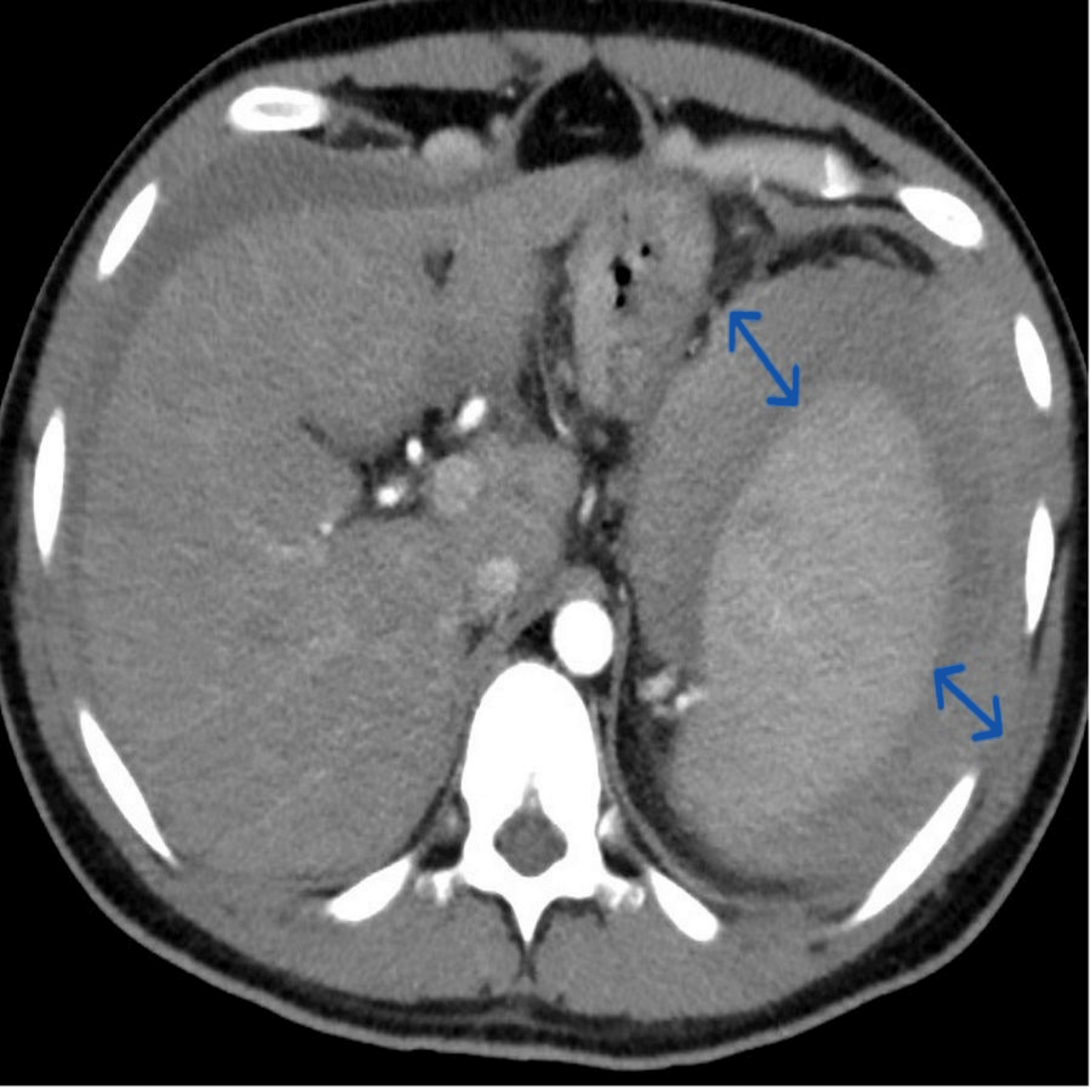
CT angiogram of the abdomen in the transverse plane showing a peri-splenic collection (blue arrows)
